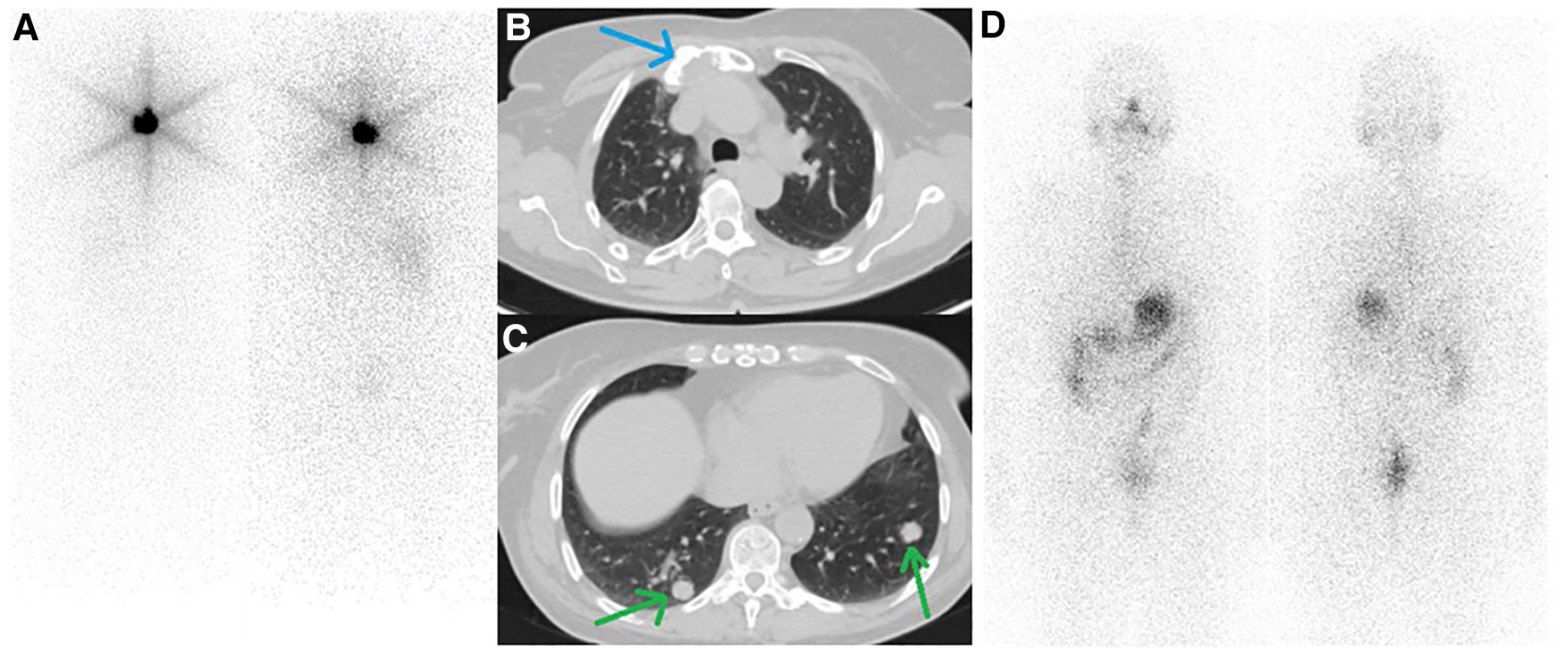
(A) First whole-body iodine scan after administration of 30 mCi of ^131^I showed post-surgical thyroid remnant. (B, C) follow-up CT scan from the thoracic region showed a lytic lesion in the sternum (blue arrow) along with multiple bilateral pulmonary nodules (green arrows). (D) The second post-treatment whole-body iodine scan after administration of 200 mCi ^131^I revealed no radioiodine-avidity in the tumoral lesion.
